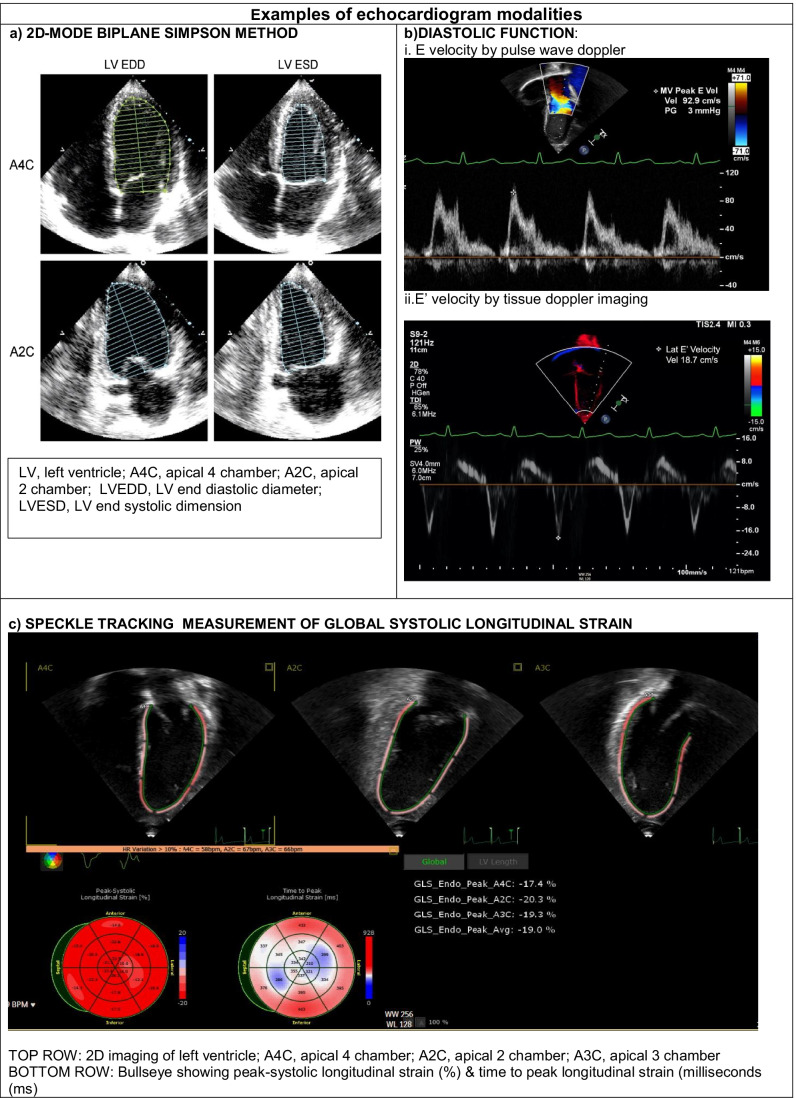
Examples of echocardiogram modalities. a 2D-mode biplane Simpson method. b Diastolic function: i, E velocity by pulse wave Doppler; ii, *E*′ velocity by tissue Doppler imaging. c Speckle tracking measurement of global systolic longitudinal strain
